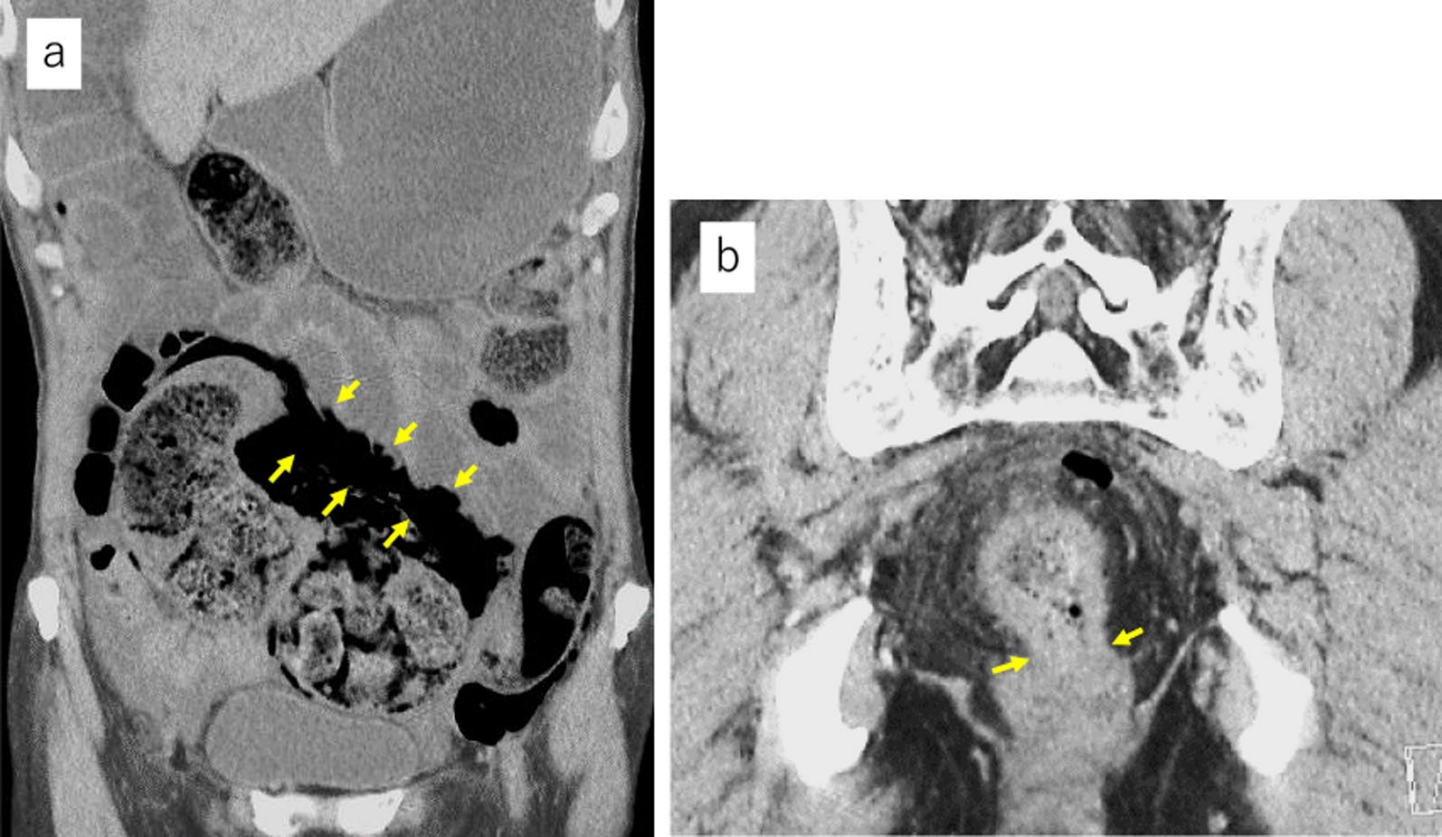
Computed tomography in case 2. a The gastrointestinal tract was dilated, and there was a large fecal mass inside the sigmoid colon with free air (yellow arrows) around it. b There was wall thickening in the rectum (yellow arrows), and the oral side of the rectum was dilated and had a fecal mass
